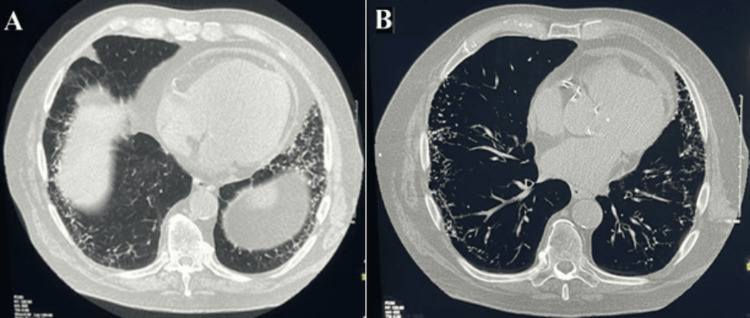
High-resolution computed tomography (HRCT) (axial view) normal window (A-B) of the chest displaying linear reticulations and interstitial thickening, with branched calcified densities seen in some areas of linear reticulation. Lung window settings (A) and bone window (B).
